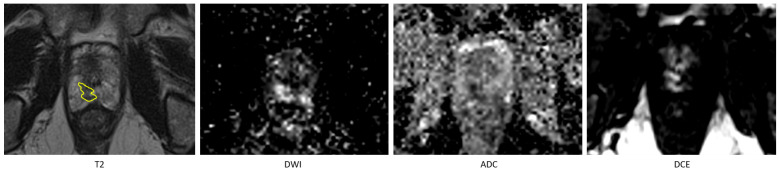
An example of missed lesion (false negative) by the fully automated AI method, where lesion is annotated by the radiologist (in yellow) on T2 hypointense area in the peripheral zone in the right apex with corresponding high signal on the high-B-value DWI and low signal on the ADC and on the DCE early enhancement.
